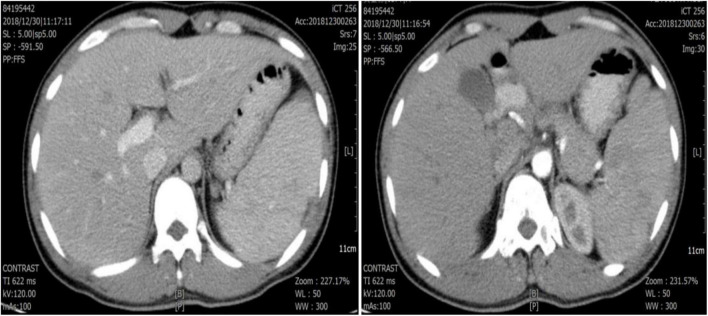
Abdominal computed tomography showed relief of splenic infarction.
